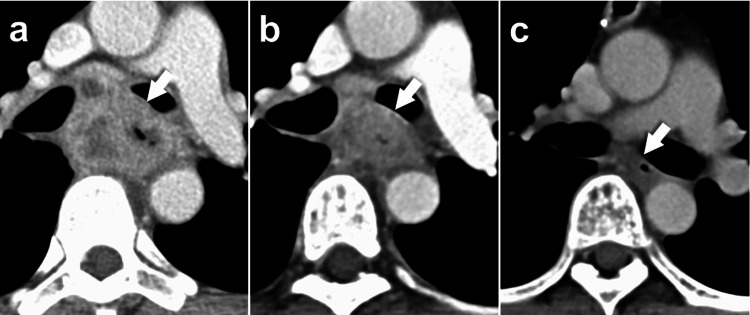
Changes in CT findings associated with treatment (a) Pre-treatment. (b) Post-DCF showing partial shrinkage but residual invasion. (c) Post-CRT and additional chemotherapy showing complete response. Arrows indicate tumor location. Chemotherapy regimen based on Hara et al. [3], and CRT protocol based on Cooper et al. [5]. CT: computed tomography, DCF: docetaxel, cisplatin, and 5-fluorouracil, CRT: chemoradiotherapy
